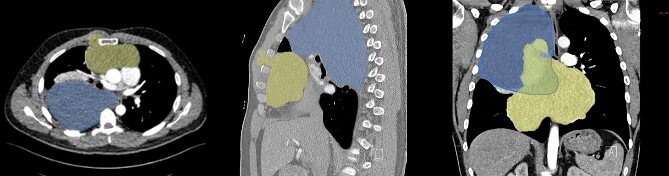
CT scan of tumor dimensions.
